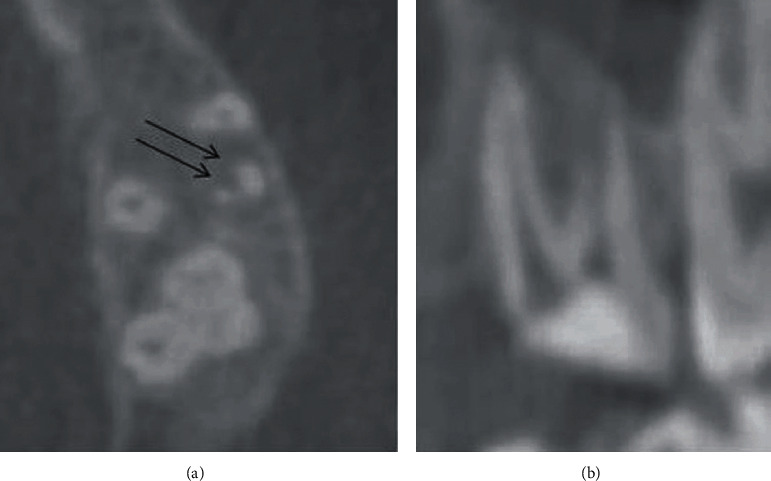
Preoperative CBCT images. The axial view of CBCT showed the apical perforation site (black arrows) at the distobuccal root (a). The sagittal view of CBCT showed the apical perforation site of the tooth apex.
